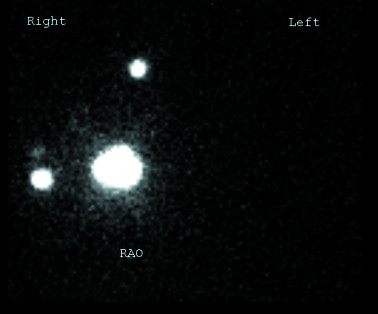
Lymphoscintigraphy demonstrating the location of the primary tumor in the upper inner quadrant of the right breast (large central area of radioactivity) as well as the two sentinel lymph nodes.
